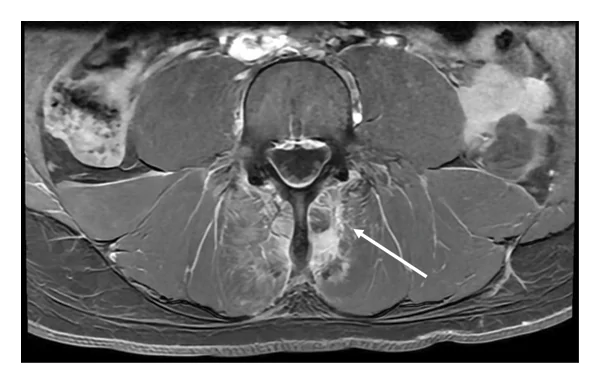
Transverse MRI of the lumbar region (a gadolinium contrast‐enhanced T1 sequence with fat saturation). The white arrow indicates peripheral enhancement of the bursa, associated with inflammation of the interspinous bursa between the third and fourth lumbar vertebrae (L3 and L4). There was no disc or bone involvement.
